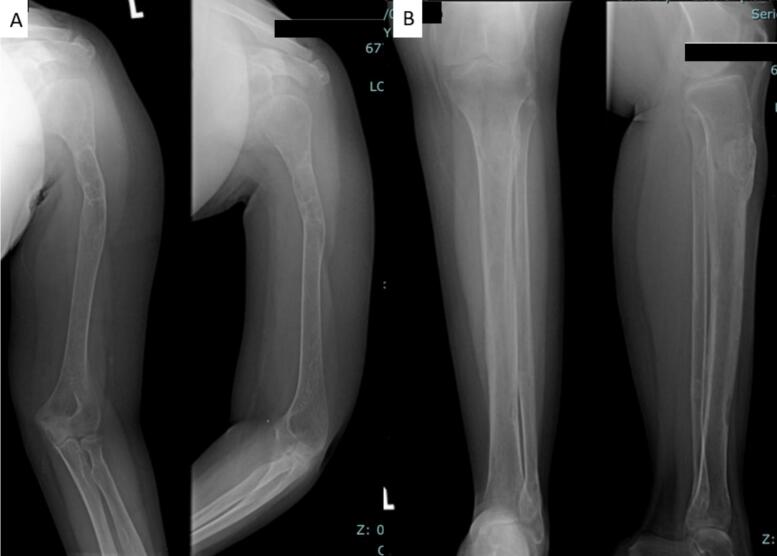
X-ray radiograph follow up 6 months post treatment hyperparathyroidism (parathyroidectomy), callus formation and thicker cortical showed regressed of brown tumor.
